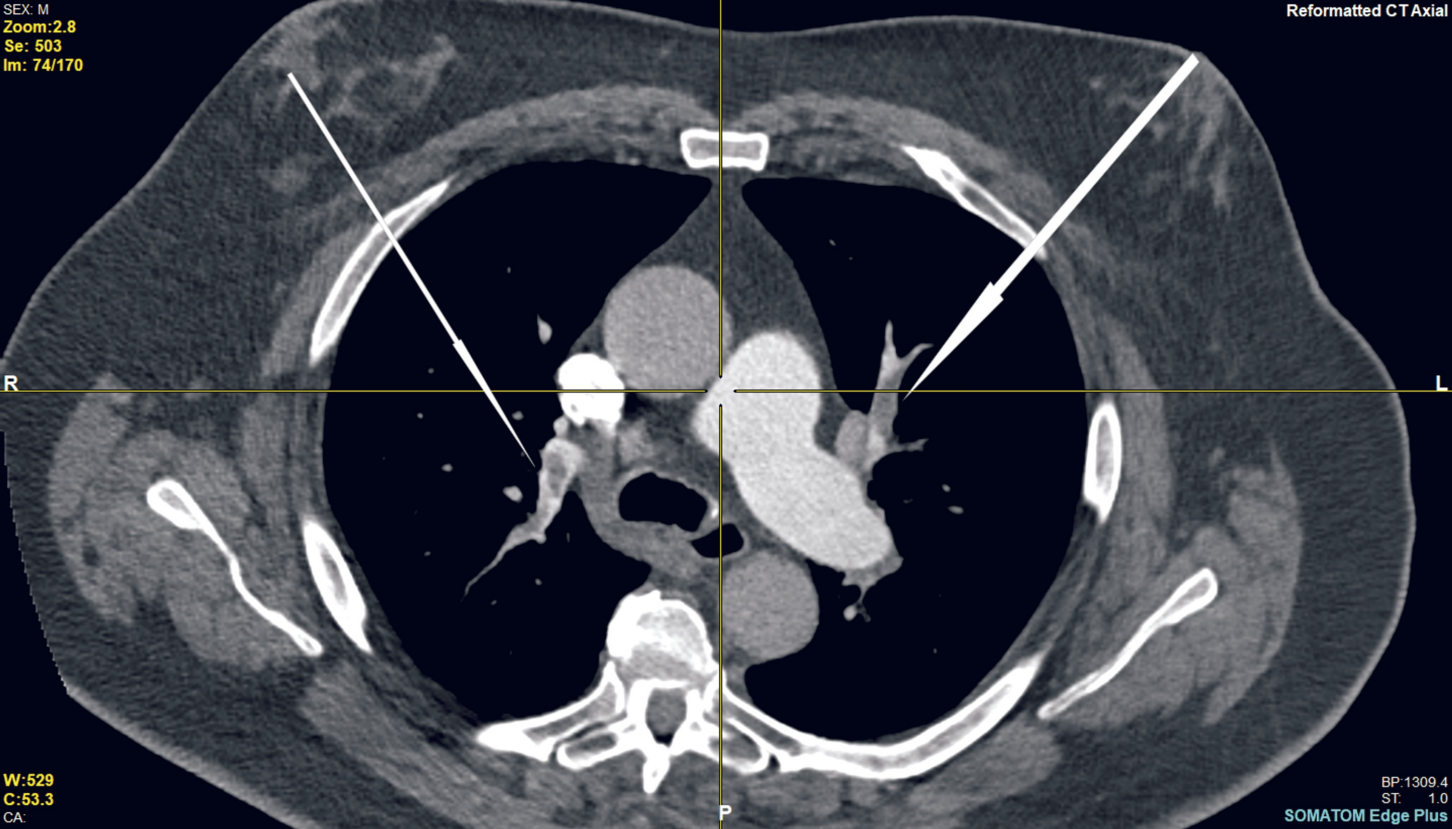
CT pulmonary angiogram, axial view (Case 2). Arrows indicate bilateral pulmonary emboli in right and left pulmonary arteries.
